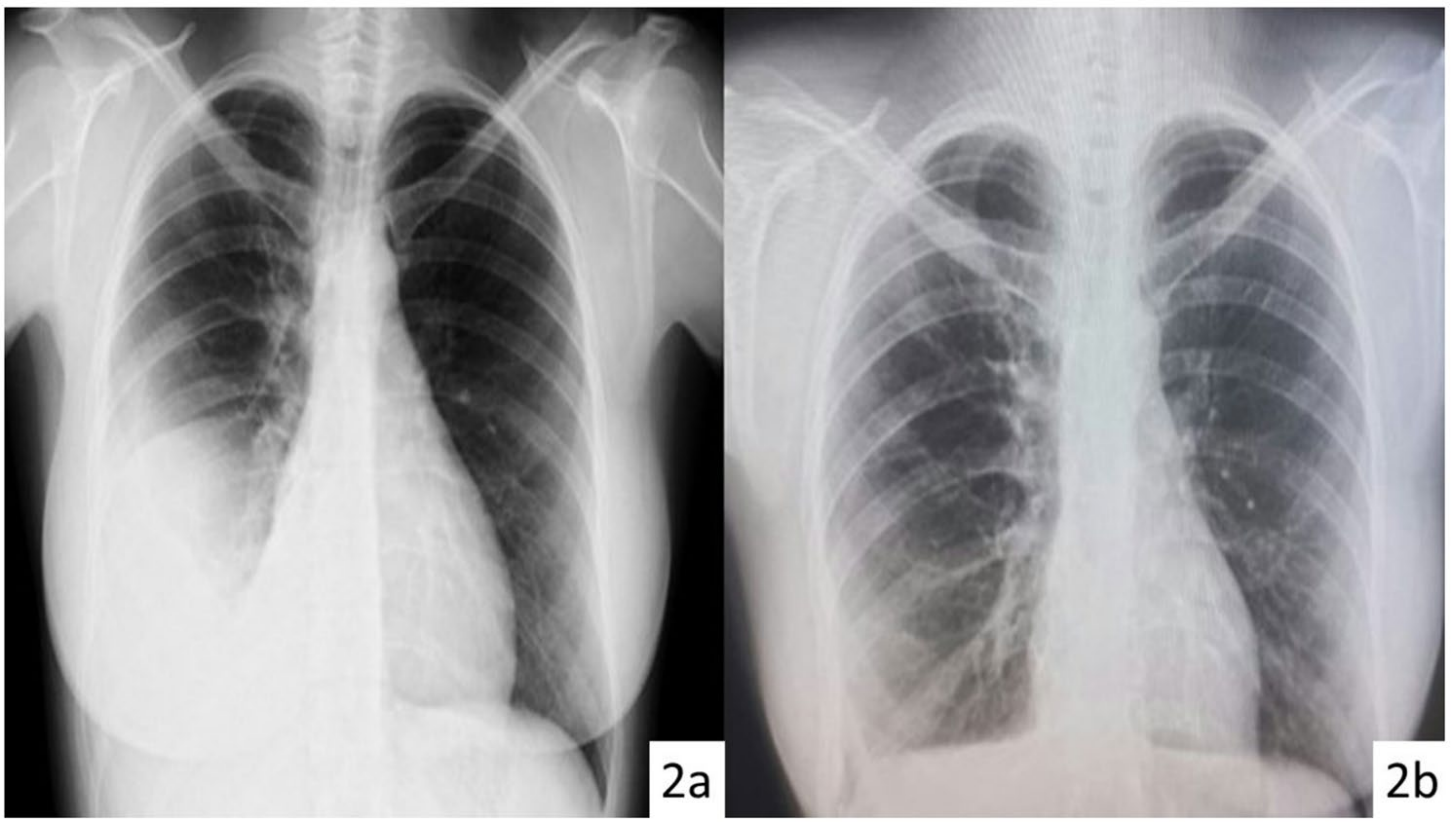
Preoperative (a) and postoperative (b) chest radiographs of a 29-year-old patient with persistent chylothorax after failed right-sided thoracic duct ligation via thoracotomy. The patient subsequently underwent transabdominal cisterna chyli ligation, resulting in spontaneous resolution of the effusion by the third postoperative month
